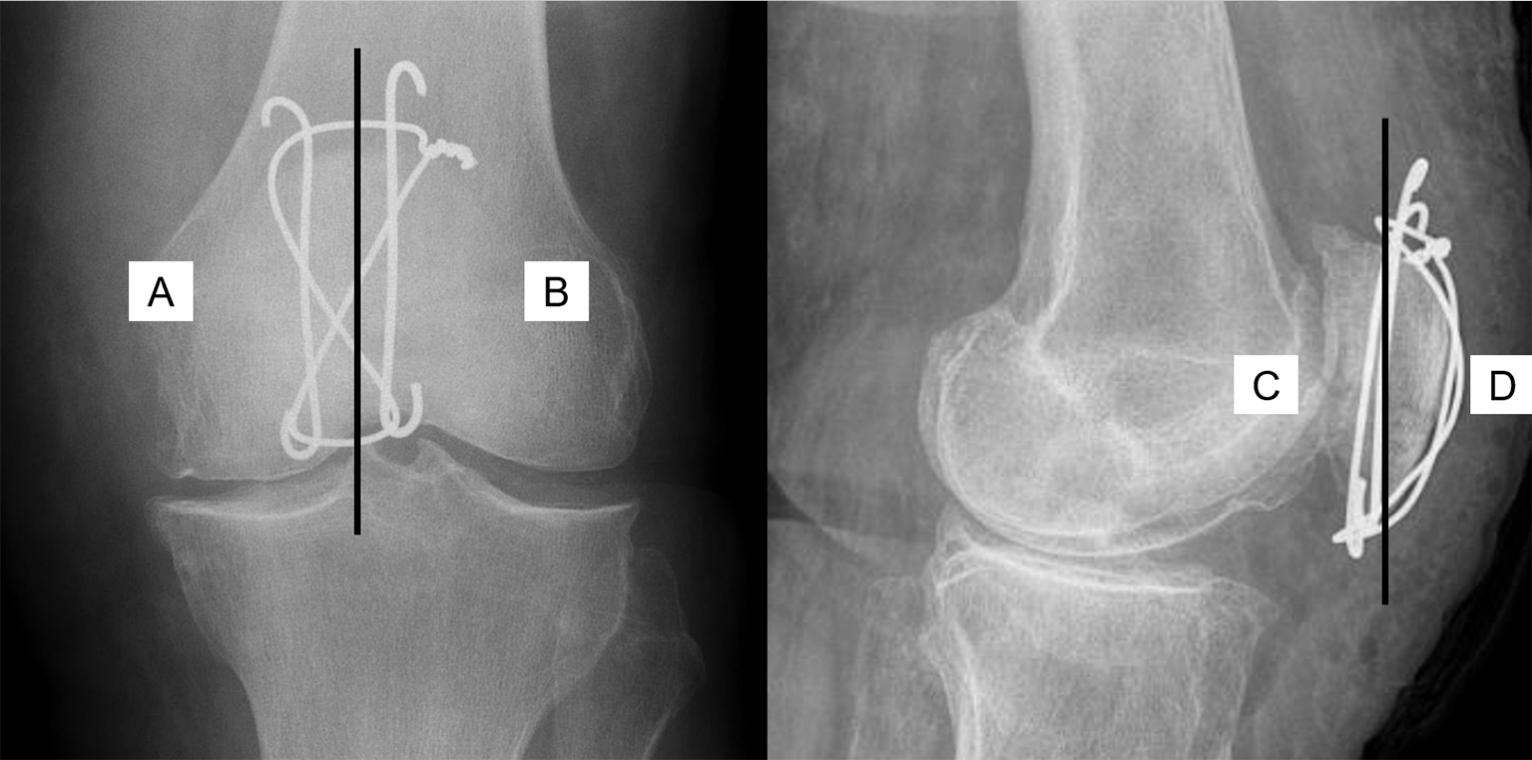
Application of the modified “Radiographic Union Score for Tibia” (RUST). A, B, C and D are to represent the individual score units
